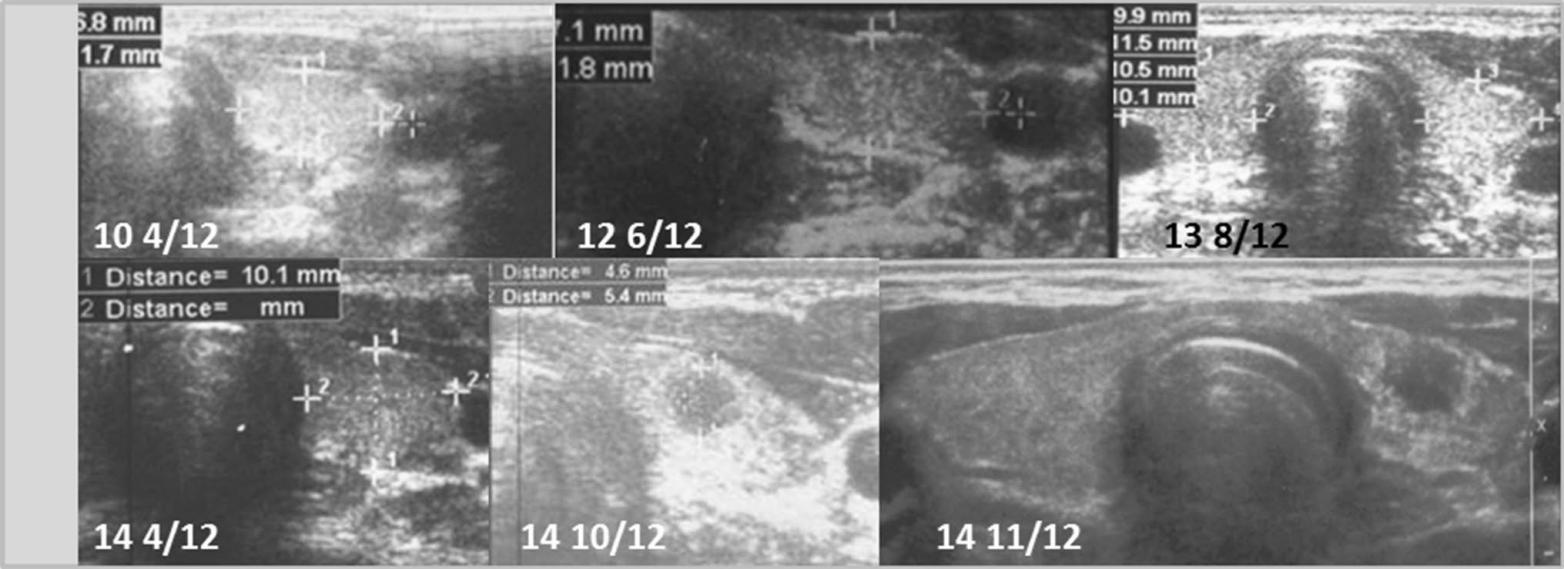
Patient 3. Transversal scans of the normoechogenic thyroid gland revealed the formation of the nodule 4 10/12 years since referral and 6 months after last ultrasound assessment. The growth of the nodule was fast from 5.4 × 4.6 × 4.6 mm to 7 × 5 × 6 mm (84% increase) in 1 month. The nodule was surrounded by a hyperechogenic border
